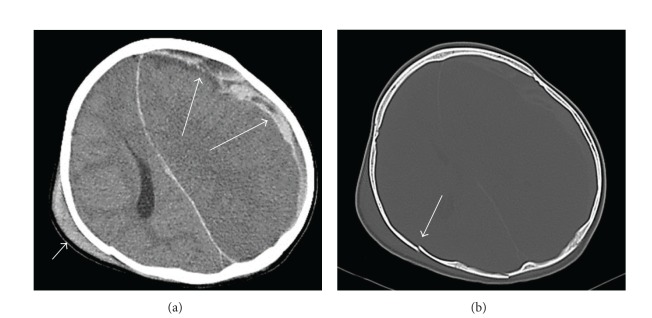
PMCT images of a 1-year-old boy with cardiopulmonary arrest. (a) Axial cranial PMCT image indicating subdural hemorrhage (long arrows) with subcutaneous hematoma (short arrow); (b) axial cranial PMCT image denoting occipital skull fracture (arrow). PMCT: postmortem computed tomography.
